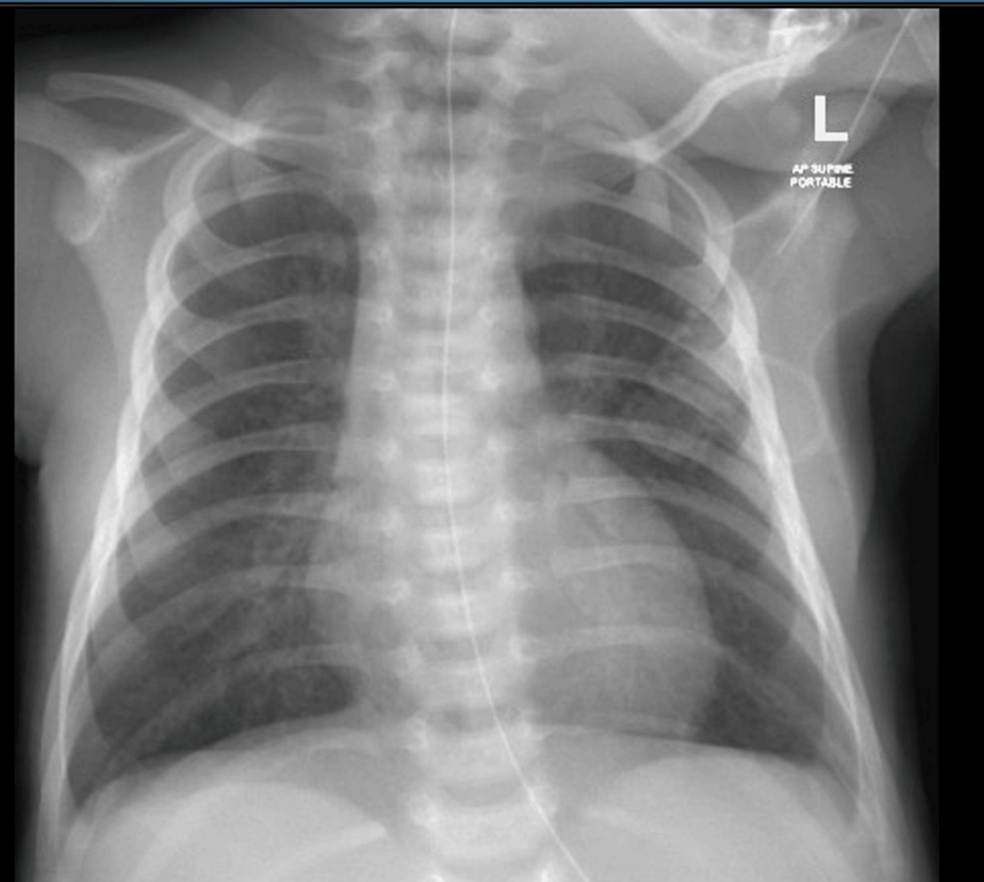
Chest X-ray before discharge
